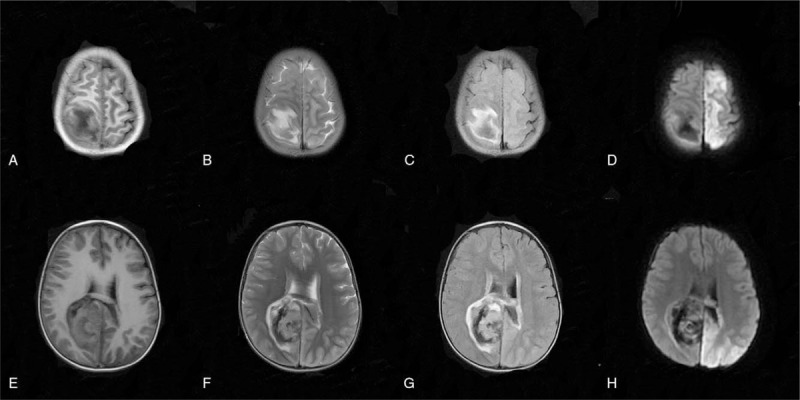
Head MRI on the 7th postoperative day. Note: A and E are T1 weighted image (T1WI), B and F are T2 weighted image (T2WI), C and G are fluid attenuated inversion recovery (FLAIR), and D and H are diffusion-weighted imaging (DWI). MRI = magnetic resonance imaging.
